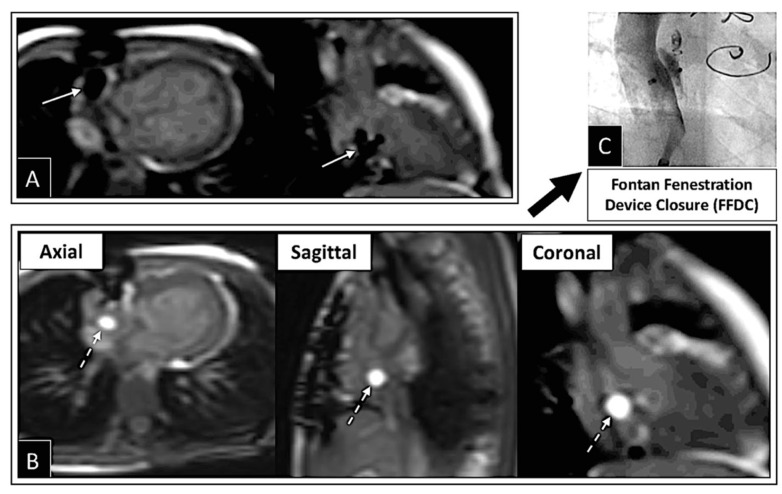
Series of images demonstrating iCMR Fontan fenestration test occlusion (FFTO) leading to device closure in the fluoroscopy lab. (A) iCMR guidance as the MR-conditional wire crosses the Fontan fenestration. Wire position is confirmed in multiple planes. (B) Multiple planes demonstrating FFTO to test eligibility for device closure. (C) Patient was deemed appropriate and transferred to the fluoroscopy X-ray suite where Fontan fenestration device closure (FFDC) was performed. Revised from ref [16].
